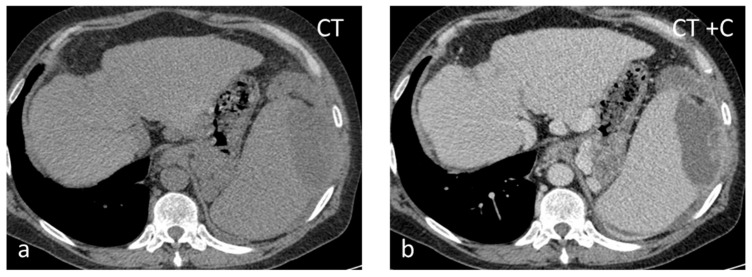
Thirty-seven-year-old male with HIV-HCV infection and splenic abscess from *E. coli*. The abscess appears as an ill-defined, subcapsular lesion with hypodense content on non-contrast CT (a) and thick irregular enhancing wall post intravenous contrast administration (b).
